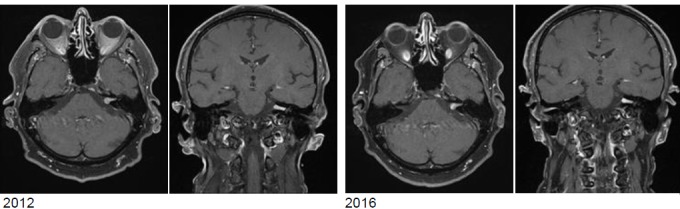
Axial and coronal MRI; T1–w + CM . Mostly constant size of a vestibular schwannoma during an observation time of 3.5 years.
